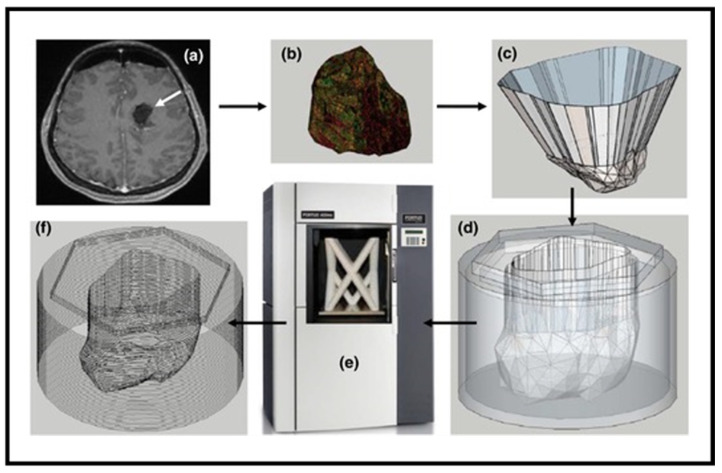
Steps to design and manufacture 3D-printed radiation device. (a) CT scan of tumor (Image credit: Dr. Tong Zhu. University of North Carolina, Chapel Hill). (b) Extraction of tumor profile. (c) Calculation of depth range data. (d) CAD (.stl format) of hollow bottle-like bolus; wall thickness tested 0.5 to 3 mm. (e) 3D printing—FDM Fortus 400 mc, ABS and PC. (f) Clinical integration of the complex bolus design irradiated at 50 Gy via electron beam therapy [41].
